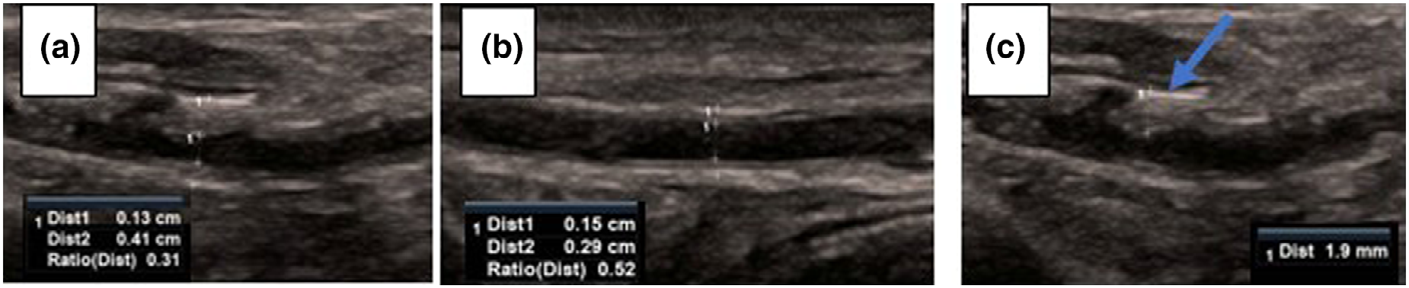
Comparison of longitudinal ultrasound image of proximal dorsalis pedis artery between a participant with rheumatoid arthritis and a control participant. (a) measured lumen and artery diameters and their ratio with areas of inflammation in participant with rheumatoid arthritis (callipers); (b) measured lumen and artery diameters and their ratio of diameters, with no evidence of inflammation in a control participant (callipers); (c) measured inflammation extending from adventitia, inflammation (callipers and arrow) in participant with rheumatoid arthritis.
